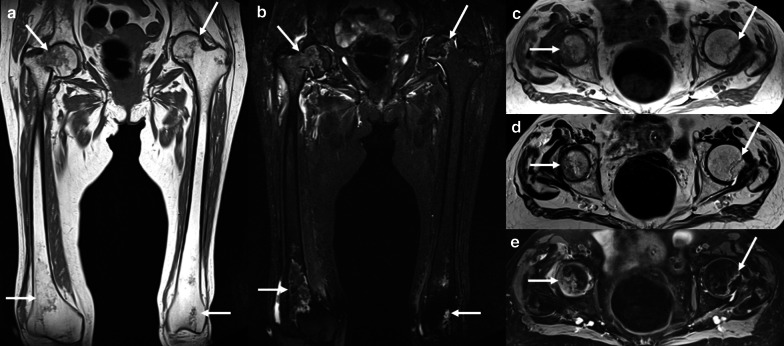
A 68-year-old man with Hodgkin Lymphoma treated with ChT (including high doses of corticosteroids) and atraumatic right hip pain. Coronal T1-weighted (a), coronal STIR (b), axial T1-weighted (c), axial T2-weighted (d), and axial proton density-weighted (e) images show geographical areas in both femurs with central fat signal, bordered by a serpiginous peripheral line, due to multifocal osteonecrosis (arrows). As a complication of osteonecrosis, a pathologic fracture of the neck of the right femur with bone marrow and perischeletric edema was identified (a, b). Also note the typical “double line sign” of the osteonecrotic area of the left femur head consisting of inner bright line (granulation tissue) and outer dark line (sclerotic bone) on axial T2-weighted image (d)
